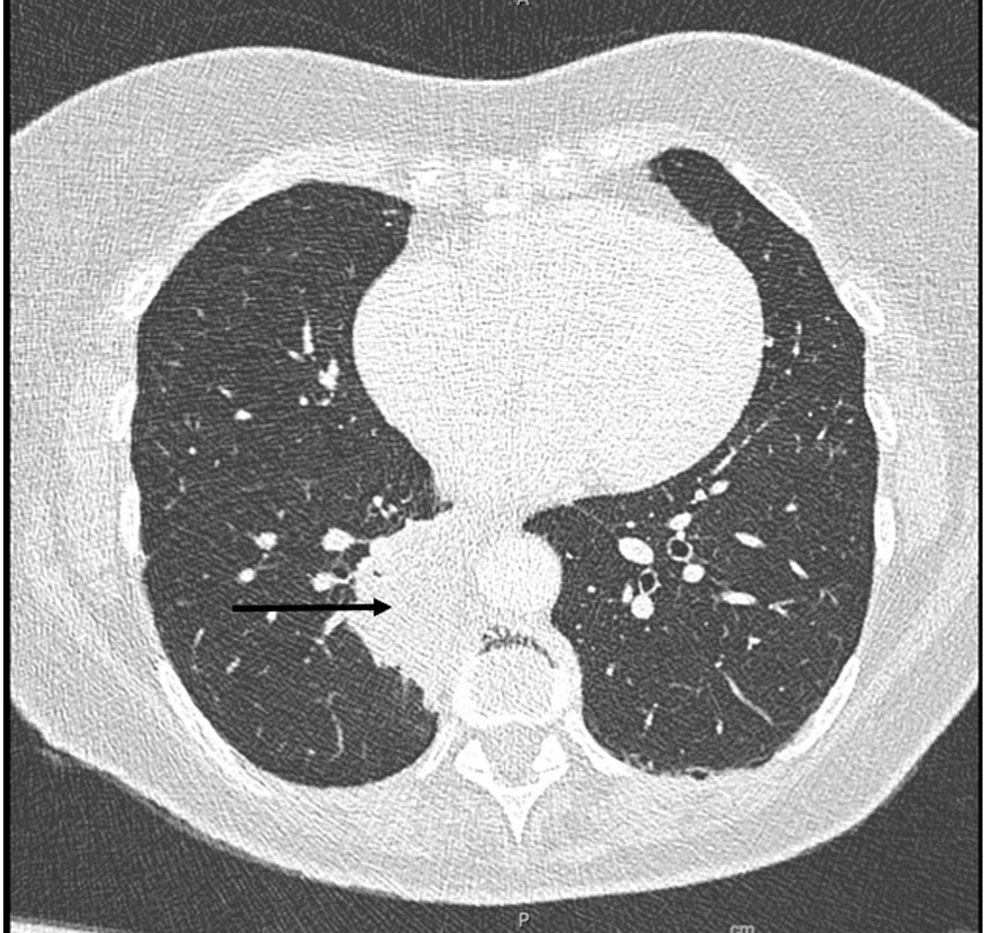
CT of chest showing a 4.1 x 2.4 x 2.6 cm heterogenous mass (arrow) in the right inferior perihilar region with associated pleural reaction and application to the T10 vertebral body
